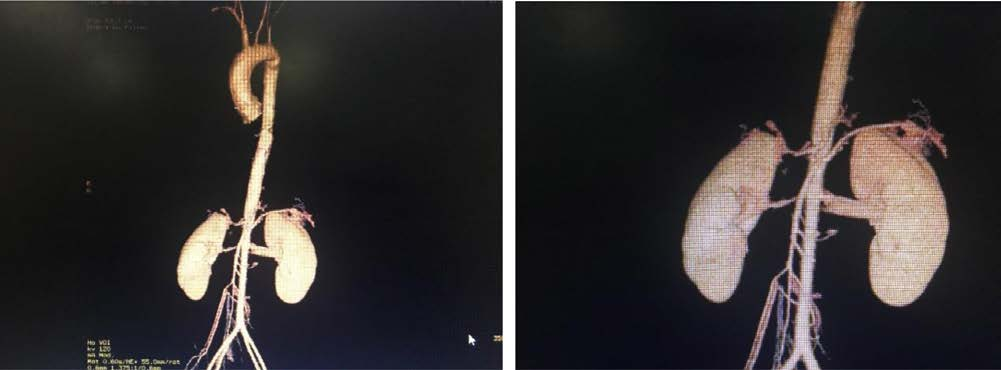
Aortic arch, thoracic aorta, abdominal aorta, left subclavian artery wall thickened, mild stenosis, right renal artery proximal severe stenosis (CTA). CTA = computer tomography angiography.
